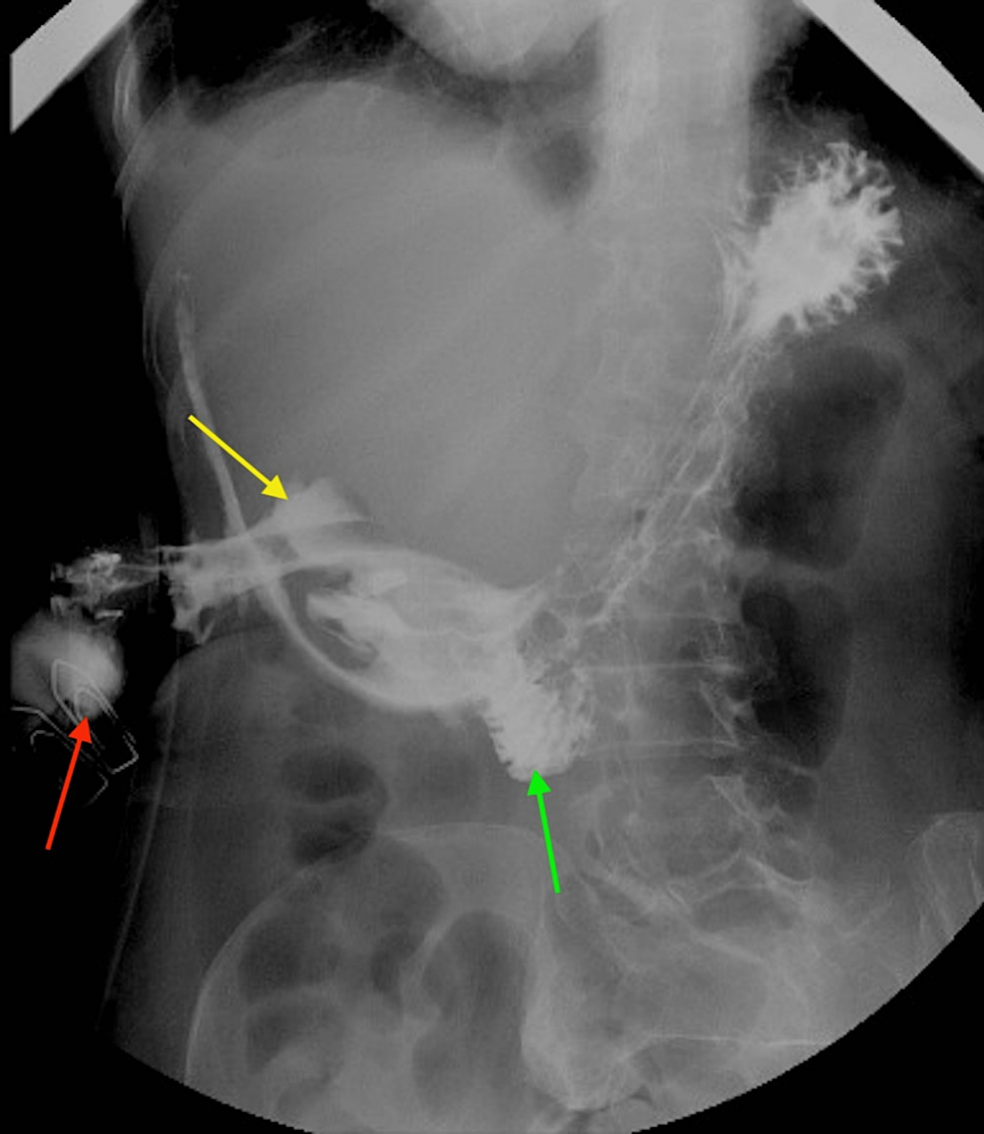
Contrast-enhanced CT - image 2 The red arrow points to the contrast injected into the Foley catheter placed in the abscess cavity. The yellow arrow points to the contrast in the subcapsular abscess cavity. The green arrow points to the contrast in the duodenum CT: computed tomography
